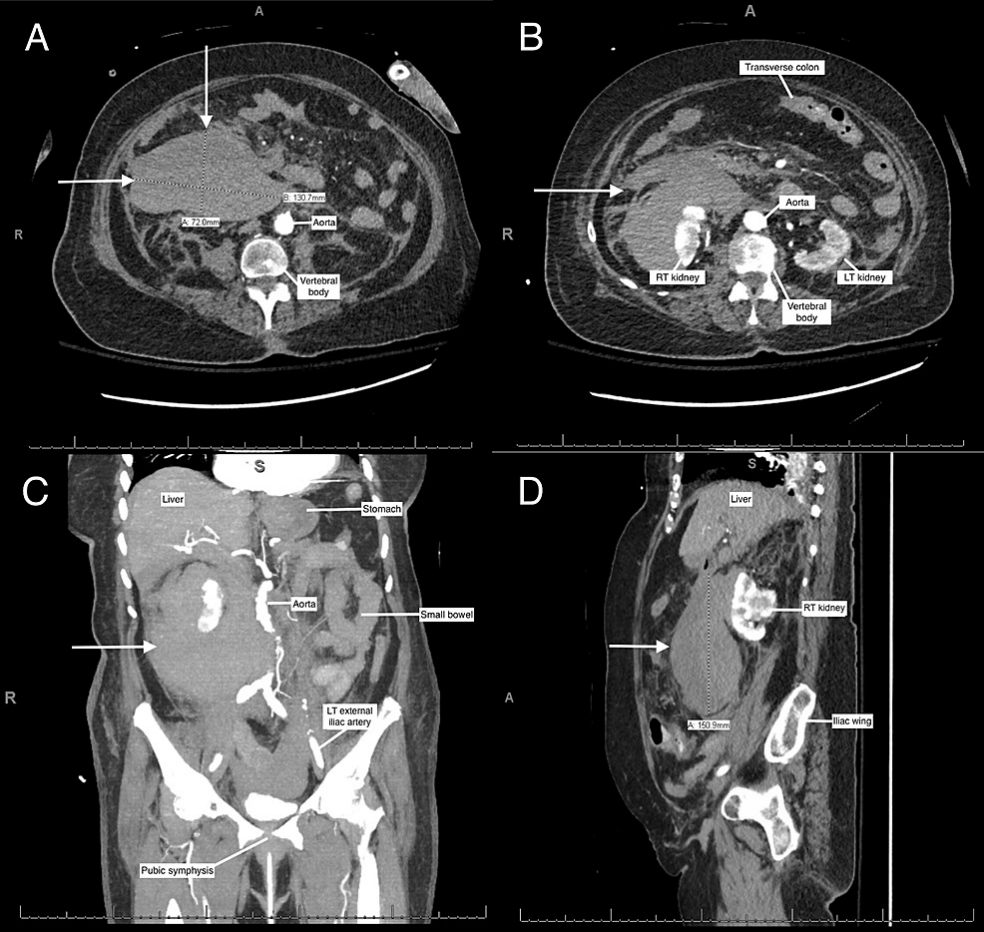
CT abdomen and pelvis of right subcapsular perinephric hematoma (A-D). CT abdomen and pelvis angiogram demonstrating a large right subcapsular perinephric hematoma measuring 7.2x13.1x15.1 cm in (A and B) axial plane, (C) coronal plane, and (D) sagittal plane (white arrows: perinephric hematoma). CT: computed tomography; LT: left; RT: right
